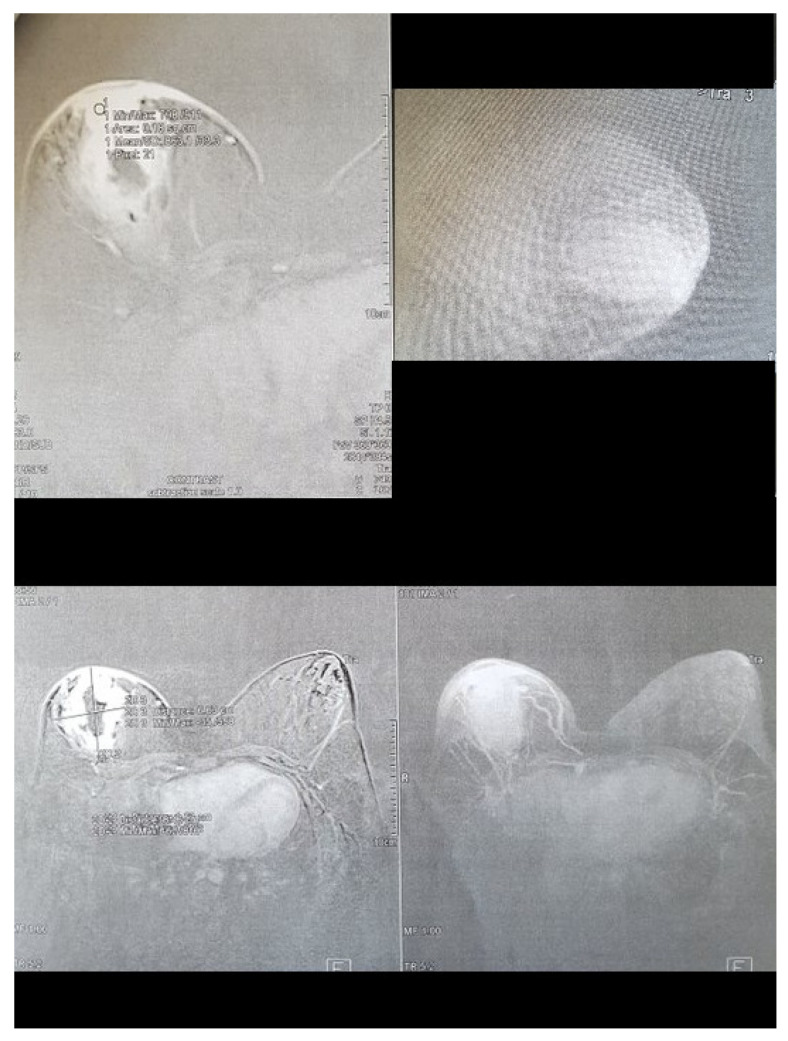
Magnetic resonance imaging of the right breast showing the following phases of the study. Visible contrast enhancement of the lesion, widening of the milk duct, and retracting the nipple.
